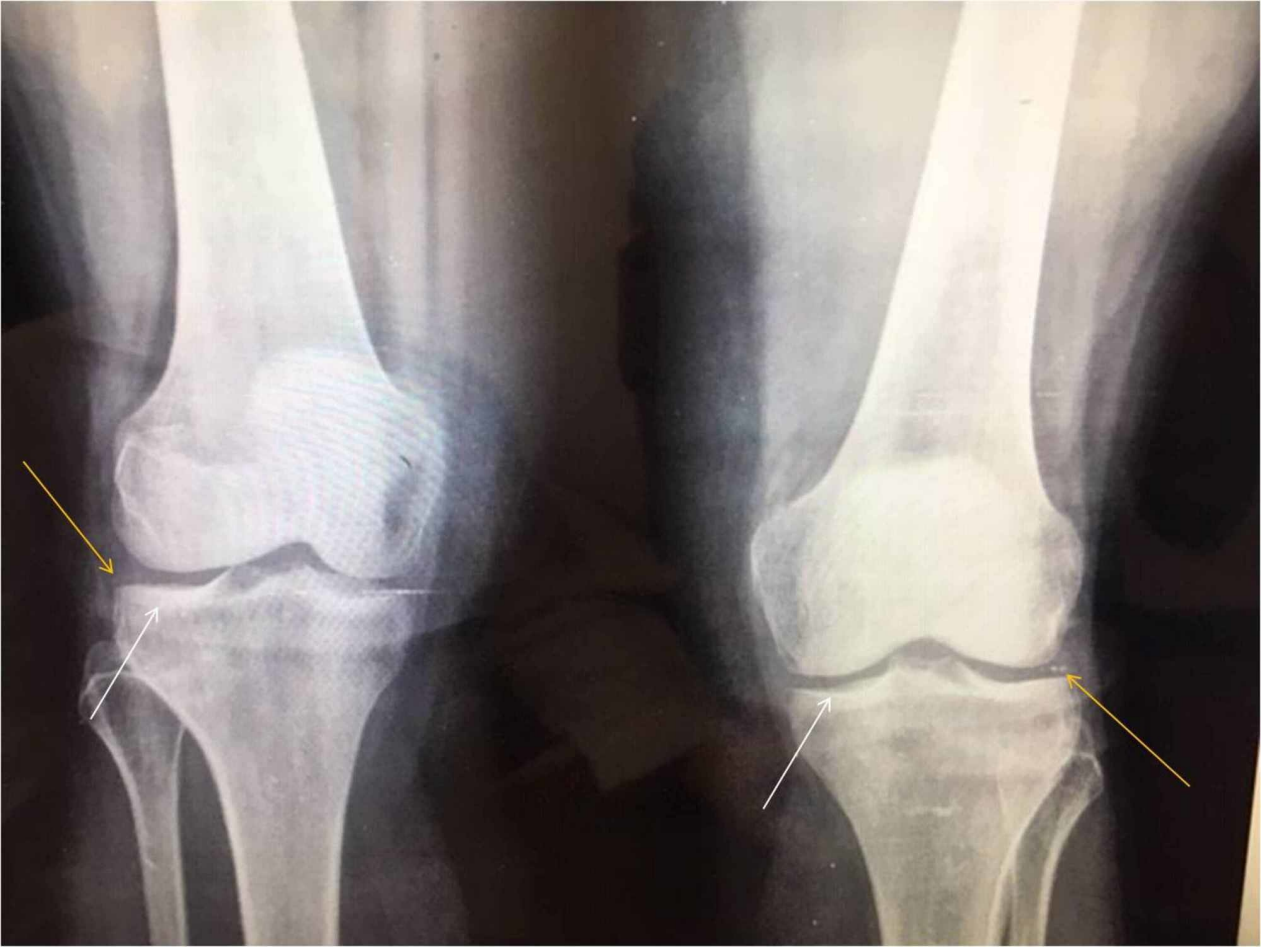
Anteroposterior X-ray views of both knees. Mild degenerative lesions with no signs of significant pathology. The white arrows point to eburnation of bone and the yellow arrows point to osteophytes and meniscal calcification.
